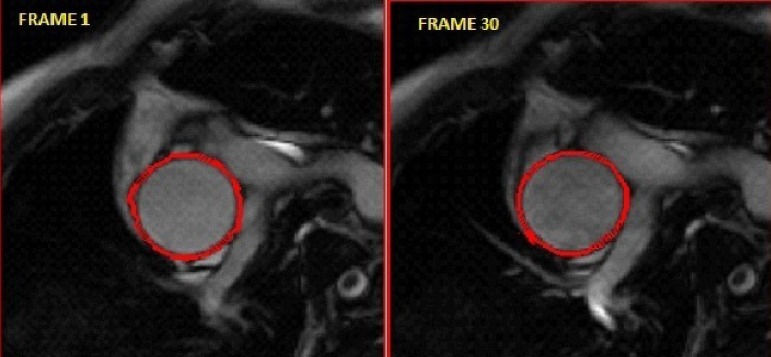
Manual processing of acquired magnetic resonance images showing first
